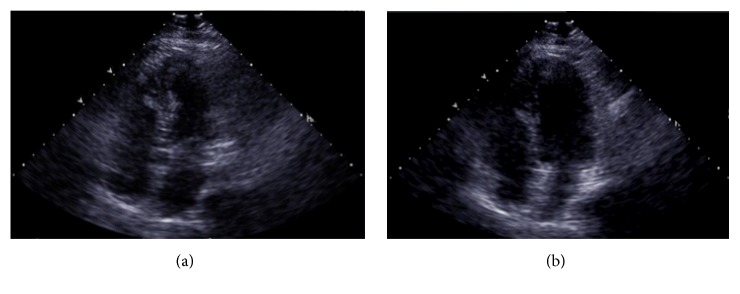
Systolic (a) and diastolic (b) apical 4 chamber transthoracic echocardiographic images. Ejection fraction estimated to be 62%. No regional wall abnormalities or valve pathology was described.
